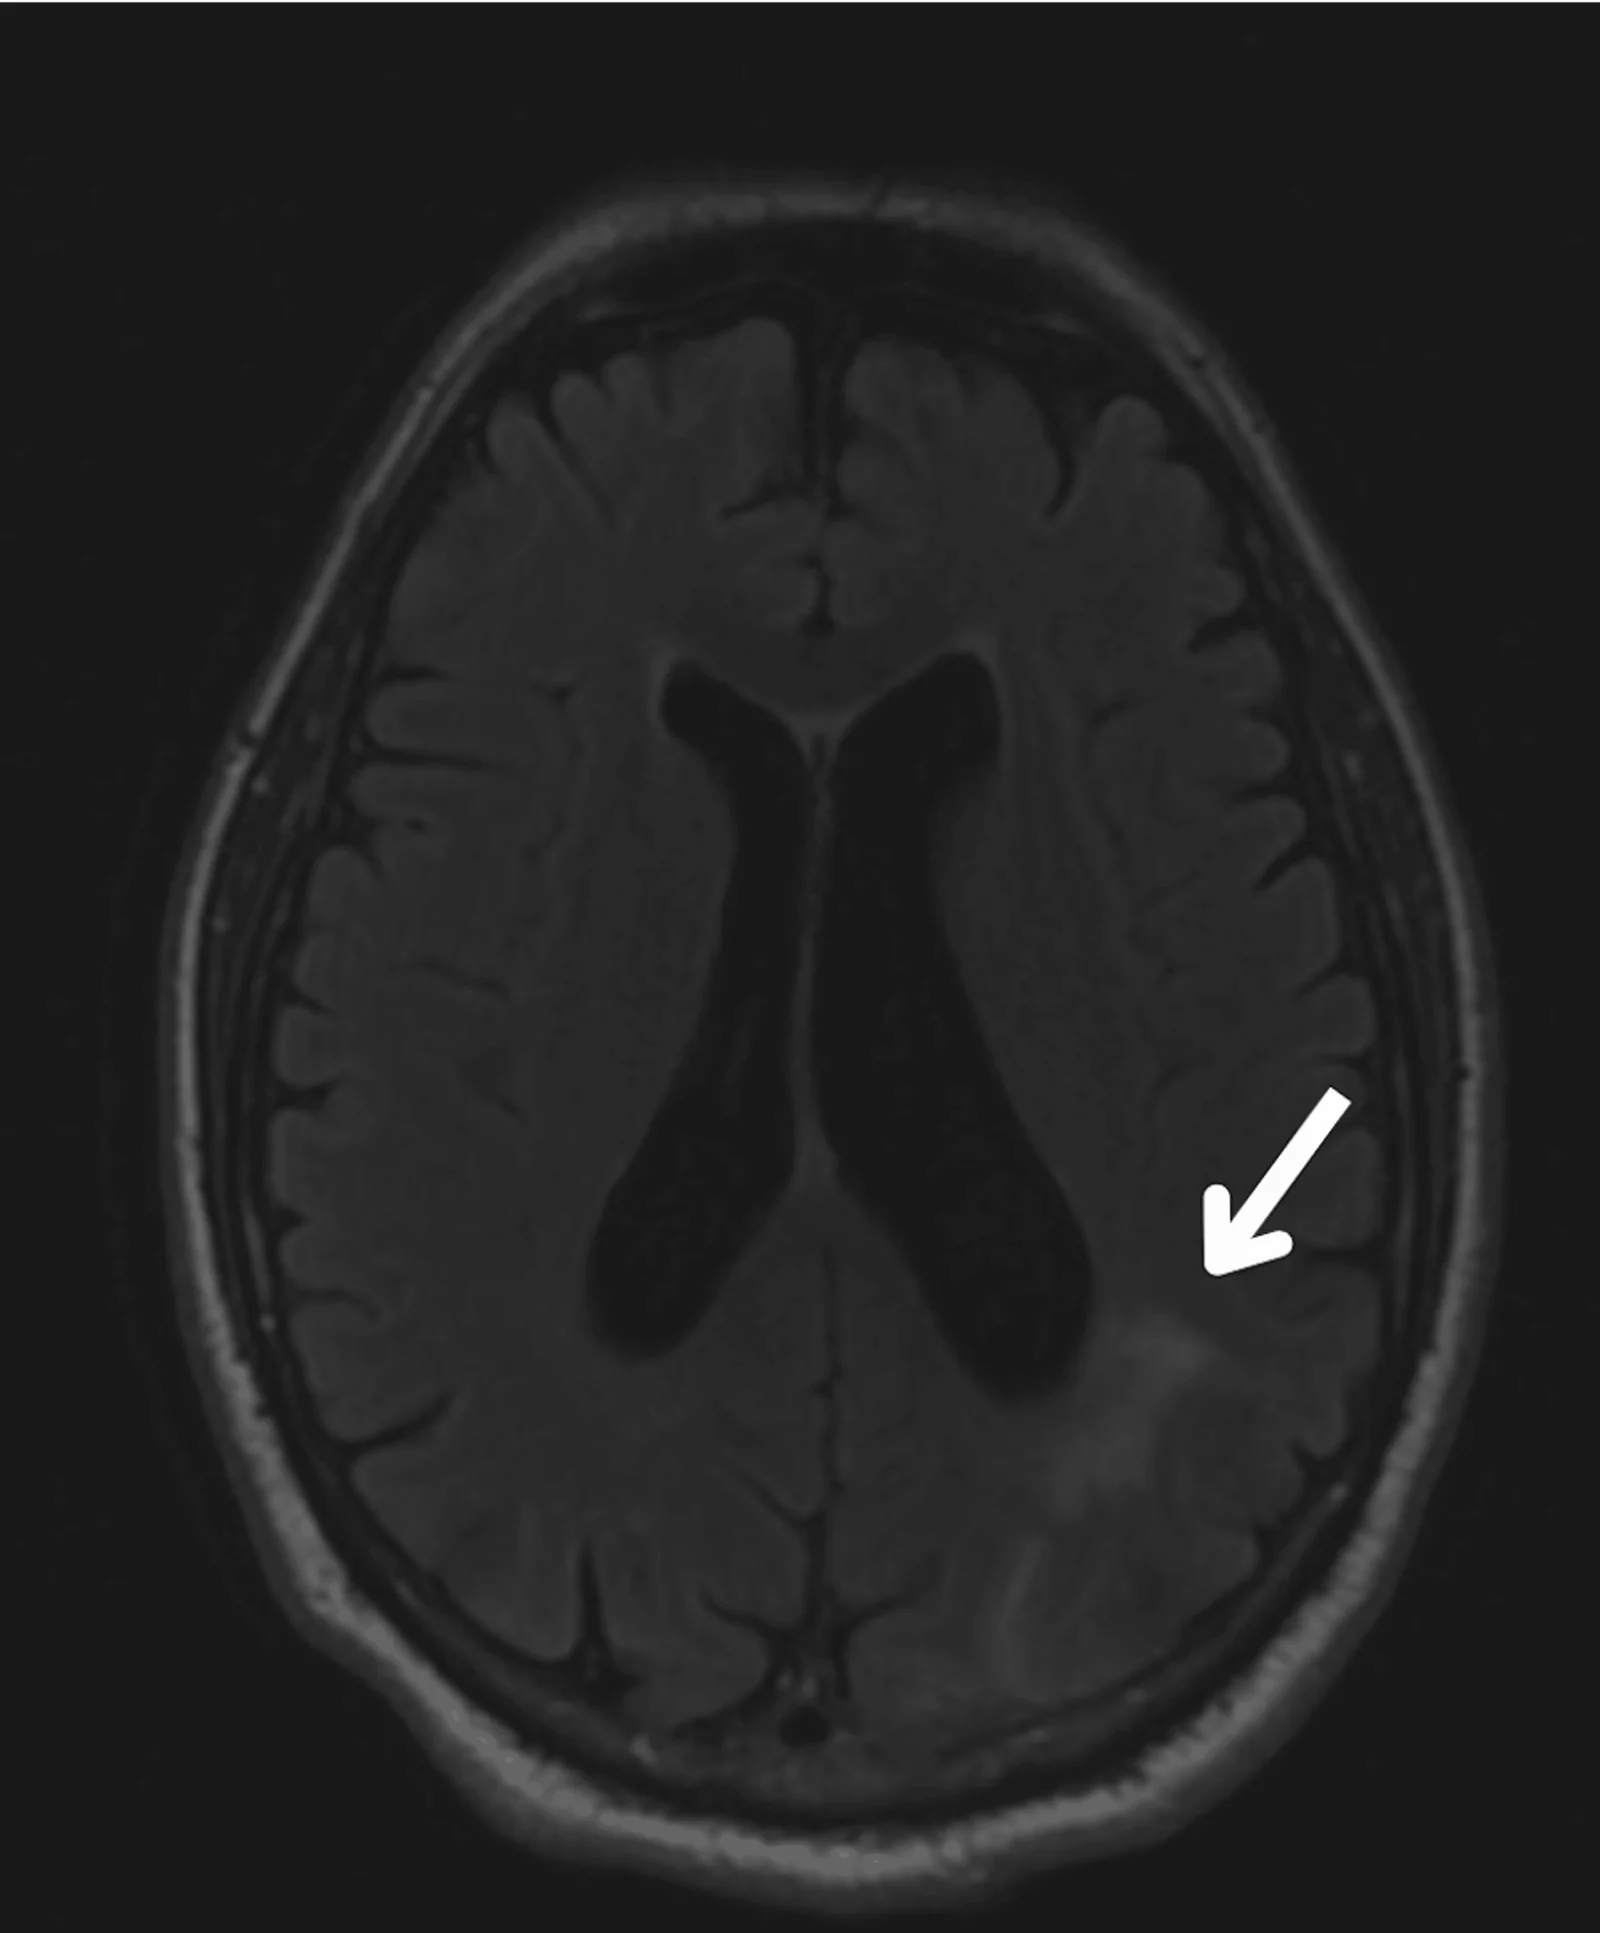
Magnetic resonance imaging (MRI) brain without contrast, fluid-attenuated inversion recovery (FLAIR) sequence Arrow demonstrating hyperintensity in the left parieto-occipital white matter, supporting the diagnosis of infarction
